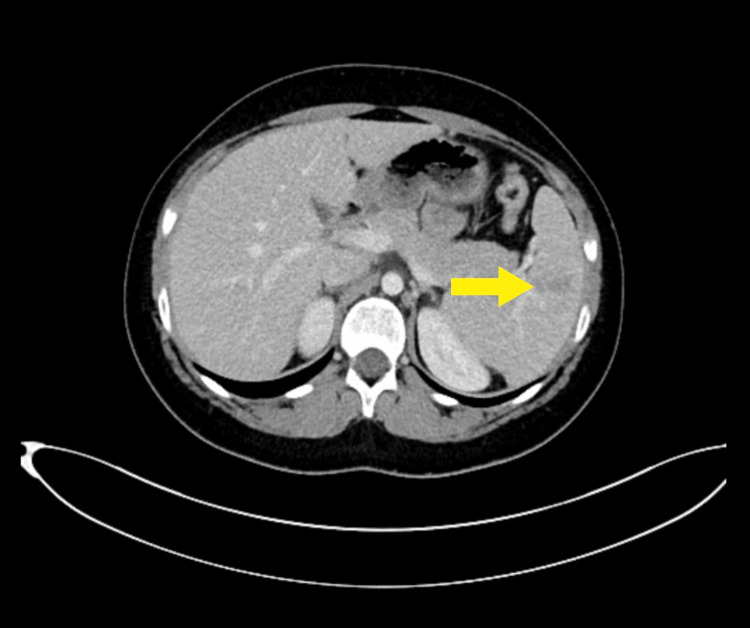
Axial view of contrast-enhanced computed tomography of abdomen suggesting grade 2 splenic lacerations showing multiple linear hypo-density extending from the splenic hilum reaching up to 2.5 cm.
